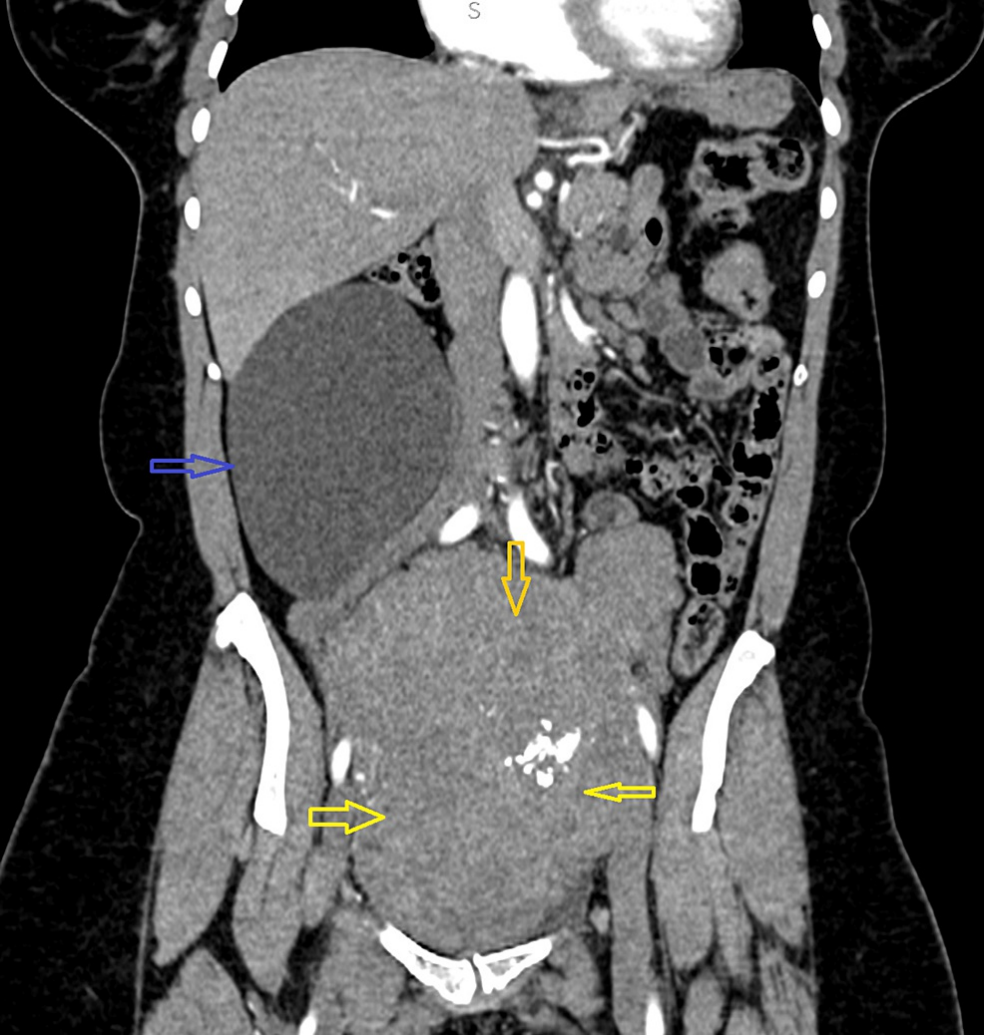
Coronal CT image of abdomen and pelvis with intravenous contrast showing the right-sided cystic lesion (blue arrow) and a large fibroid uterus (yellow arrows) CT, computed tomography
